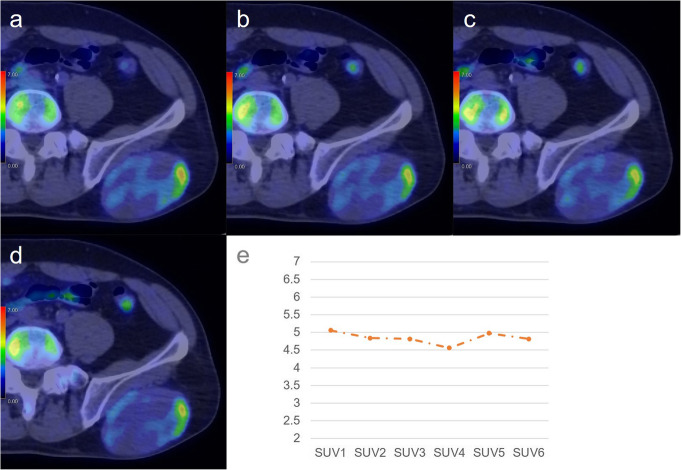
A 59 year-old-man with pleomorphic leiomyosarcoma (grade 2) in the lower back. Dynamic positron-emission tomography/computed tomography (PET/CT) images are shown for the dynamic phases (a–d). In the dynamic first phases (a), the methionine accumulation in soft tissue tumours is inhomogeneous, and the focal intense accumulation is identifiable in the left portion of the tumour (SUVmax, 5.06). After the dynamic second phase (b–d), the focal intense accumulation gradually decreased (SUVmax: dynamic second phase (b), 4.84; dynamic third phase, 4.82; dynamic fourth phase (c), 4.56; dynamic fifth phase, 4.98; dynamic sixth phase (d), 4.82). The SUVmax remained relatively stable with minimal fluctuation during the dynamic phases (e)
